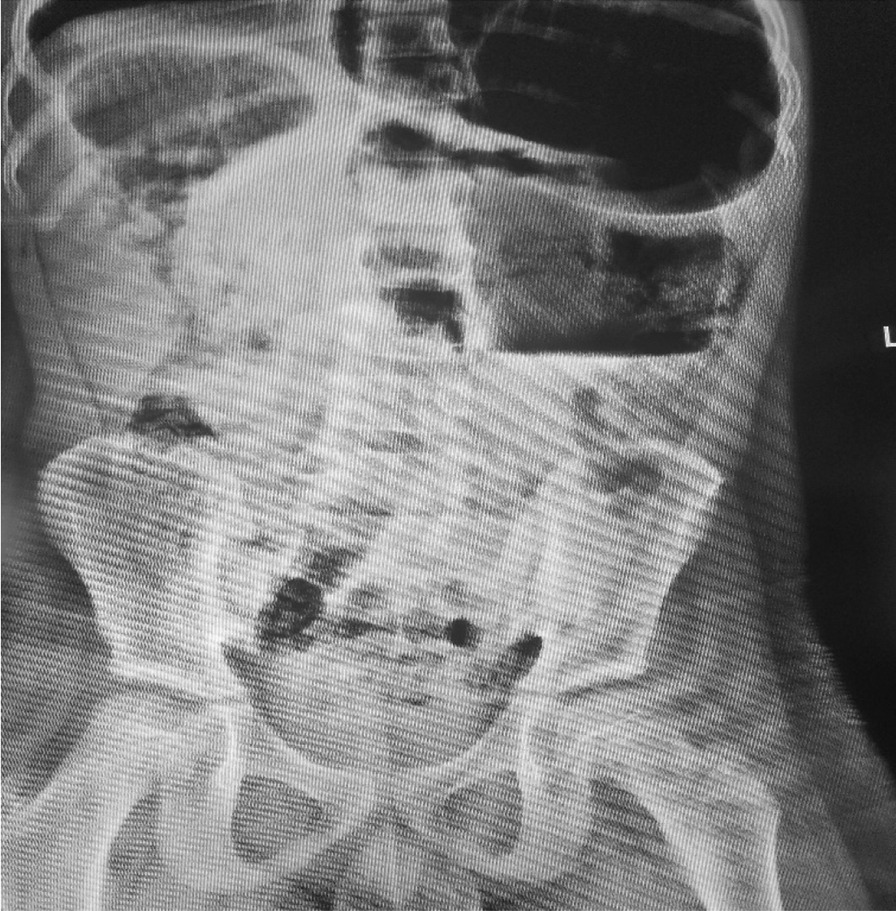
PA plain abdominal x-ray showing single air fluid level with coffee bean appearance
